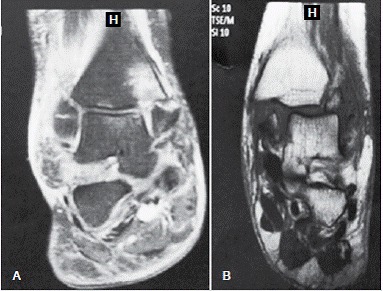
(A) Magnetic resonance imaging of the ankle in coronal plane showing a Weber B fracture, supination-external rotation stage 4, with fragment of the medial malleolus containing a small deviation and integrity of the deltoid ligament. (B) Magnetic resonance imaging of the ankle in coronal plane showing a Weber B fracture, supination-external rotation stage 4, with medial malleolus fragment 2.3 cm in size and total rupture of the deltoid ligament.
